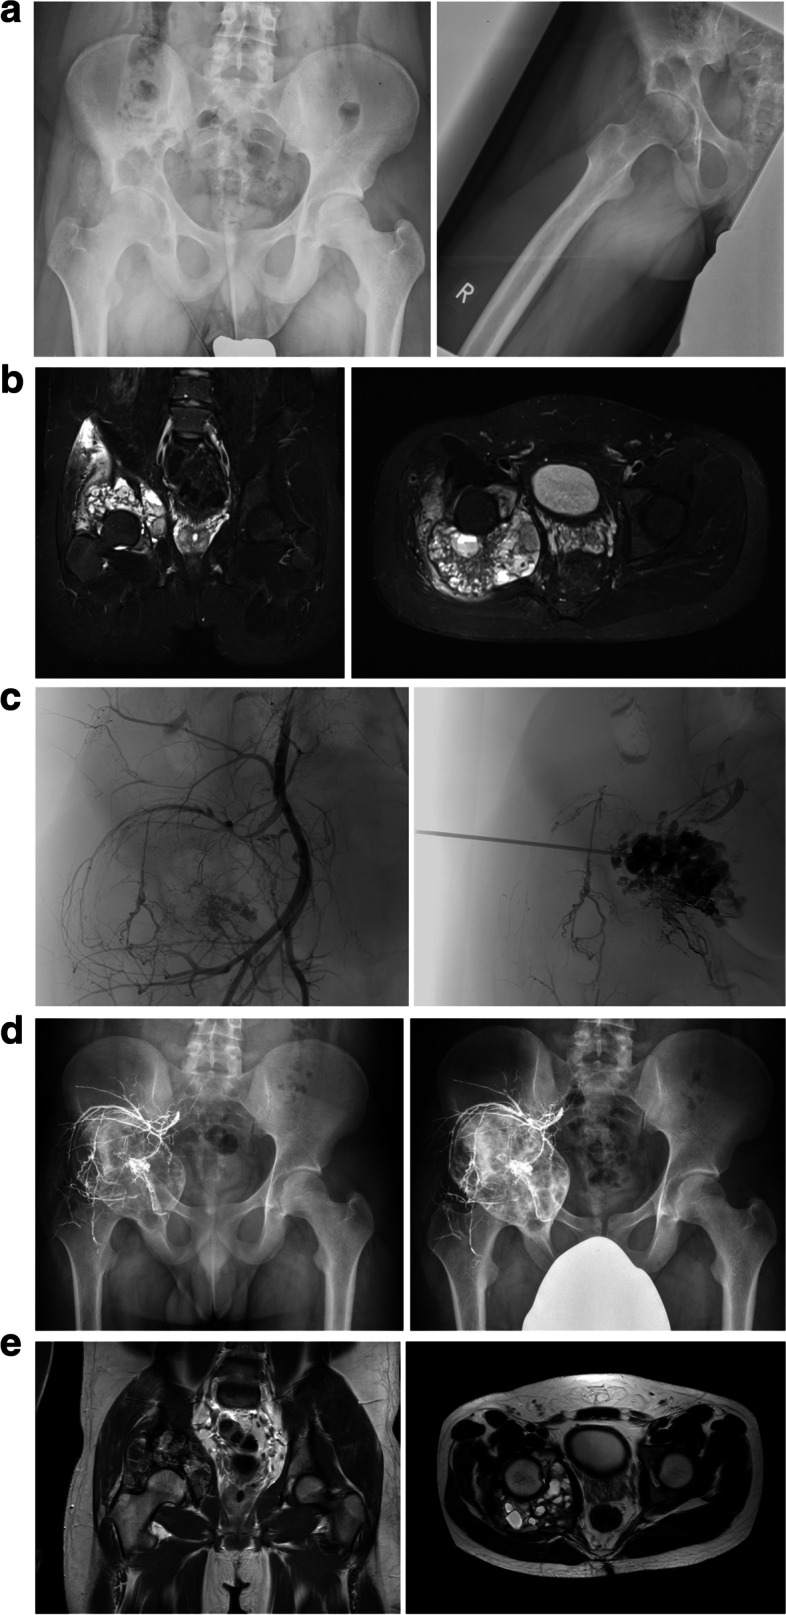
a-e ABC of the right ilium of a 15-year-old patient: simple radiograph (a); MRI scan (b); transarterial embolization and percutaneous sclerotherapy (c), simple radiographs 6 weeks (left) and 24 months after the sixth and last instillation (right; d); MRI scan 24 months after the last sclerotherapy with constant residual cystic elements (e)
